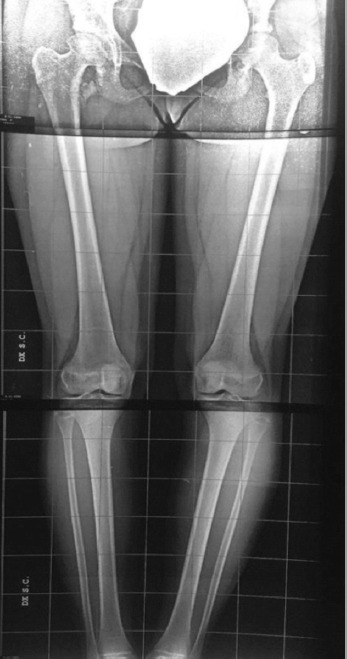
Underloading lower limb radiograph, made four years after the injury, demonstrating total healing of the greater trochanter, a dysmorphic right femoral head and no leg length discrepancy.
